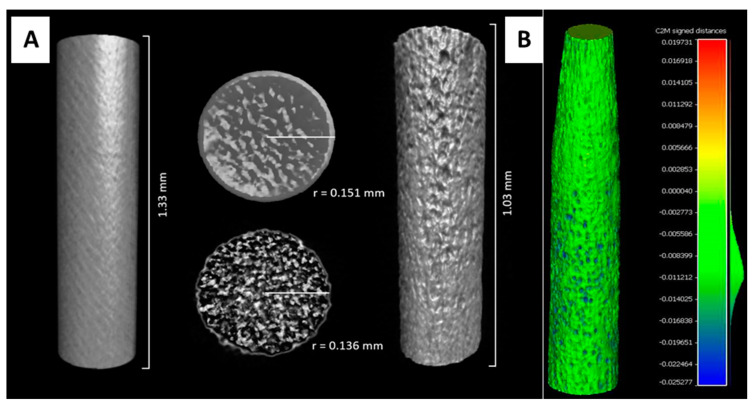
Micro CT images generated with CTVOX software (A) and Cloud Compare software (B). mage A depicts UAN vs. PAN5 change in diameter as a result of pitting corrosion, with material loss of 0.015 mm. Image B confirms the material loss across the entire PAN5 surface, following a normal distribution ranging from 0.08–0.011 mm.
